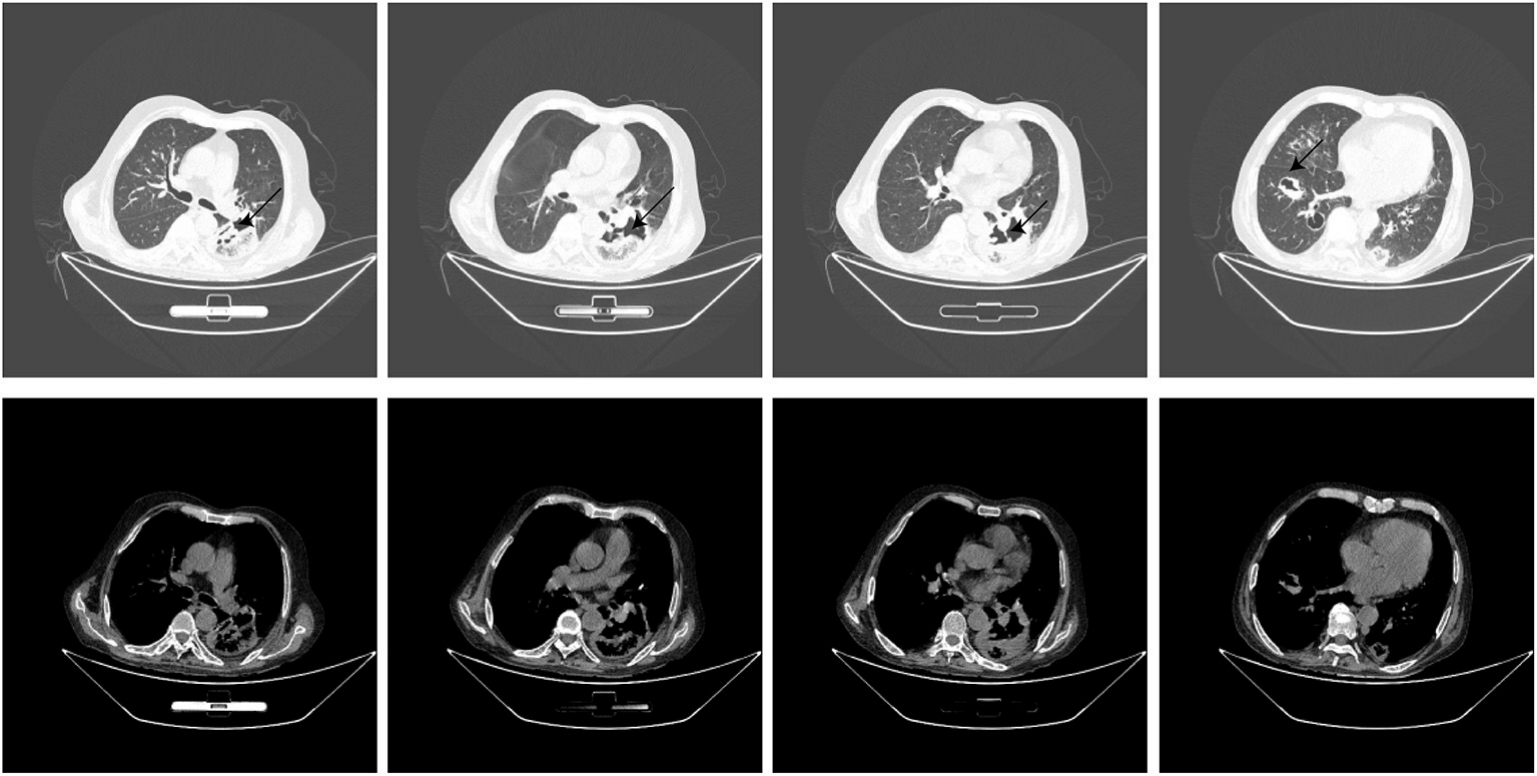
Chest CT 10 days after transfer.
